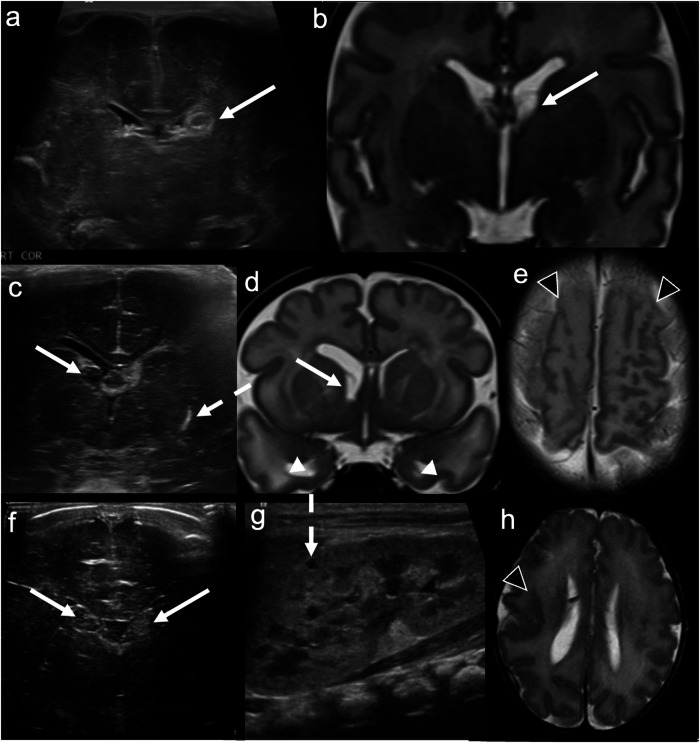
Caudothalamic groove subependymal/germinolytic pseudocysts. Post-haemorrhagic subependymal cyst. a Coronal image acquired with a high-frequency (10–15 mHz) linear probe at the level of the frontal horns demonstrates echogenic, expansile material in the left frontal horn, with central hypoechogenicity (solid arrow). Appearances are consistent with grade 1/subependymal haemorrhage with evolving cystic change. b Subsequent coronal T2-weighted MRI at the same level demonstrates a simple cyst in the left frontal horn (solid arrow), at the site of the prior subependymal haemorrhage. Congenital cytomegalovirus (CMV) infection. c Coronal image acquired with a high-frequency (10–15 mHz) linear probe at the level of the frontal horns demonstrates a cyst in the right frontal horn (solid arrow) in addition to left lenticulostriate mineralising vasculopathy (dashed arrow). d Subsequent coronal T2-weighted MRI at the same level demonstrates a simple right frontal horn cyst (solid arrow), as well as bilateral temporal pole cysts (white arrowheads). e Axial T2-weighted MRI towards the vertex demonstrates an abnormal, nodular morphology of bilateral frontal lobe gyri, consistent with polymicrogyria (black arrowheads). This constellation of findings—periventricular cysts (especially in the anterior temporal poles), lenticulostriate mineralisation and polymicrogyria—is suggestive of congenital CMV infection, which was proven in this patient. Zellweger syndrome. f Coronal image acquired with a high-frequency (10–15 mHz) linear probe at the level of the frontal horns on day 1 of life demonstrates bilateral echogenic, expansile frontal horn cysts (solid arrows), slightly less echogenic than expected for acute subependymal haemorrhage. g Sagittal renal ultrasound image in the same patient demonstrates a tiny renal cortical cyst (dashed arrow). h Axial T2-weighted MRI at the level of the lateral ventricles demonstrates nodularity of the right peri-Sylvian cortex consistent with polymicrogyria (black arrowhead). This constellation of findings—germinolytic and renal cortical cysts and polymicrogyria—is typical of Zellweger syndrome, which was proven in this patient
